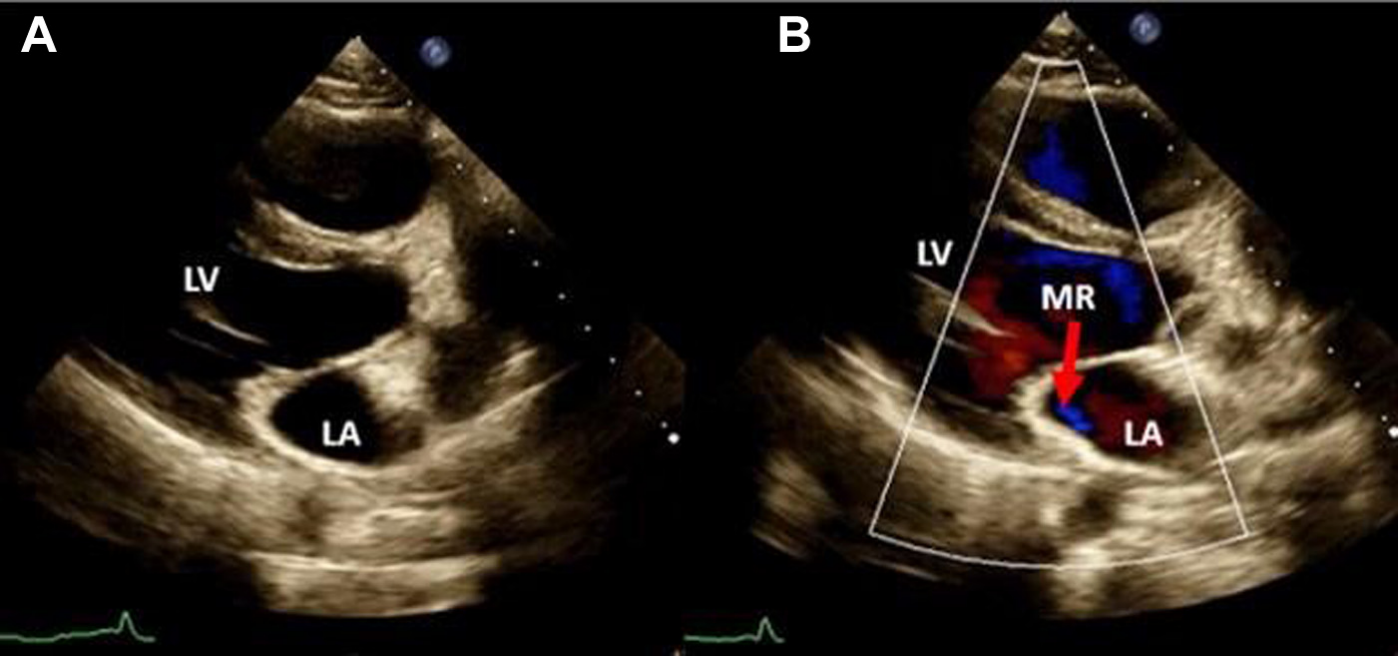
Two-dimensional TTE parasternal long-axis view, systolic phase without (A) and with (B) color flow Doppler, demonstrates mild eccentric, laterally directed, mitral regurgitation (MR; *arrow*). *LA*, Left atrium; *LV*, left ventricle.
